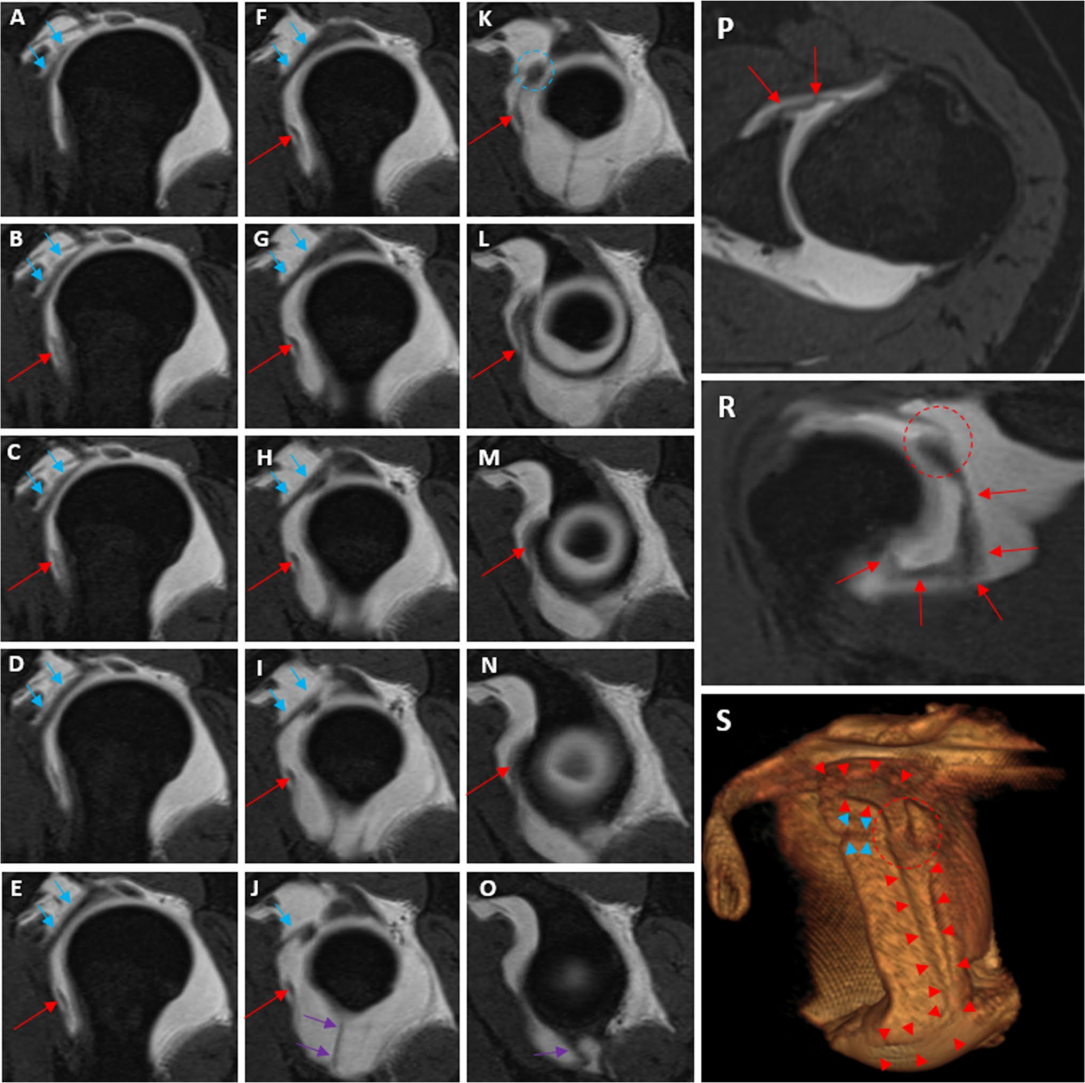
Consecutive sagittal oblique A–O, axial P, coronal oblique R, and 3D volumetric rendering S VIBE T1-weighted MR arthrograms demonstrate clearly SpiGHL (red arrows and red arrowheads) conjugating (circle in frames K, R, and S) with MGHL and other ligamentous structures such as SGHL (blue arrows and blue arrowheads) and AB-IGHL (purple arrows)
